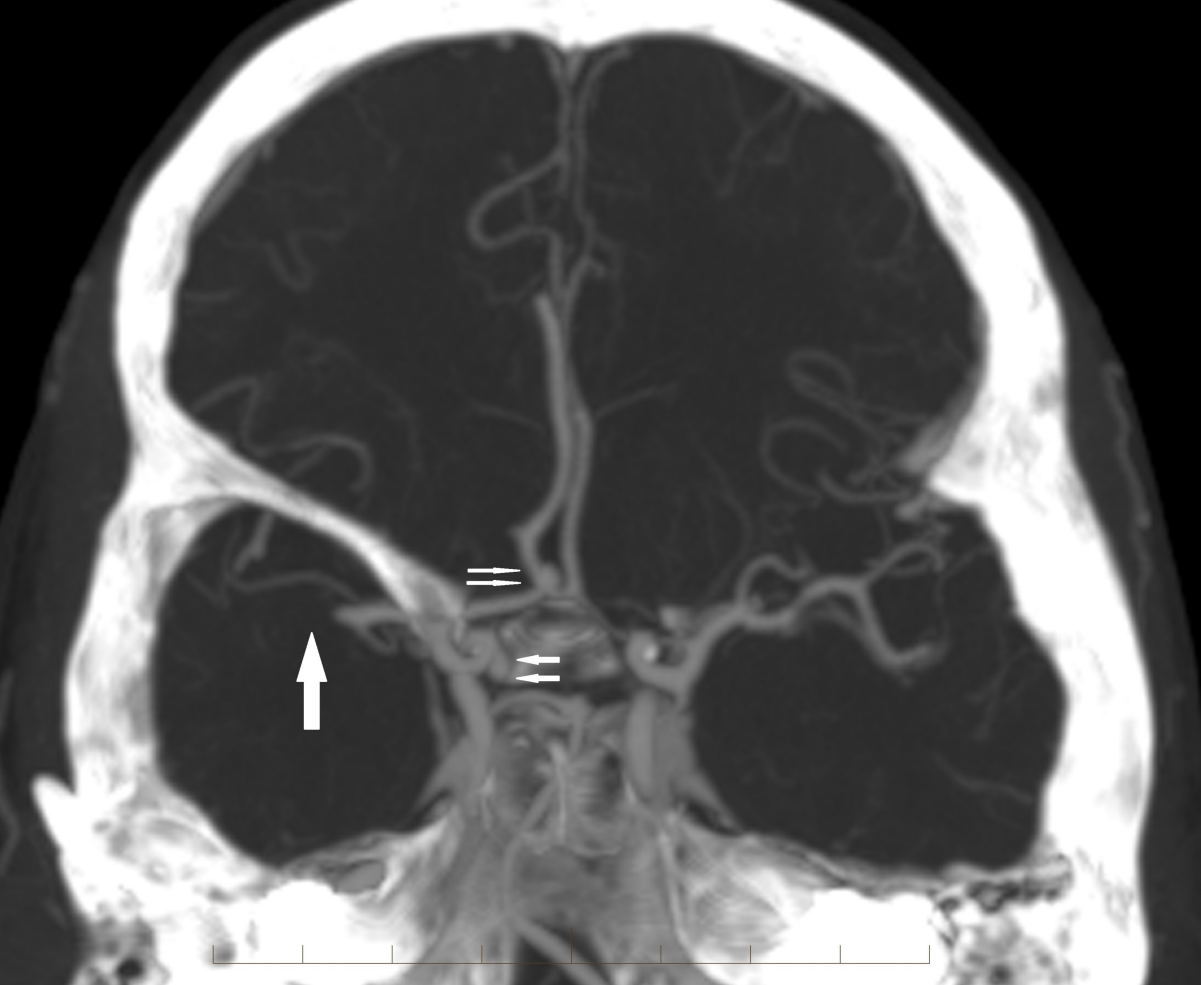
Coronal reconstructed image from the computed tomography angiogram confirms the right M1 segment middle cerebral artery occlusion (arrow). Also noted are the right superior hypophyseal and anterior communicating artery aneurysms (double arrows)
